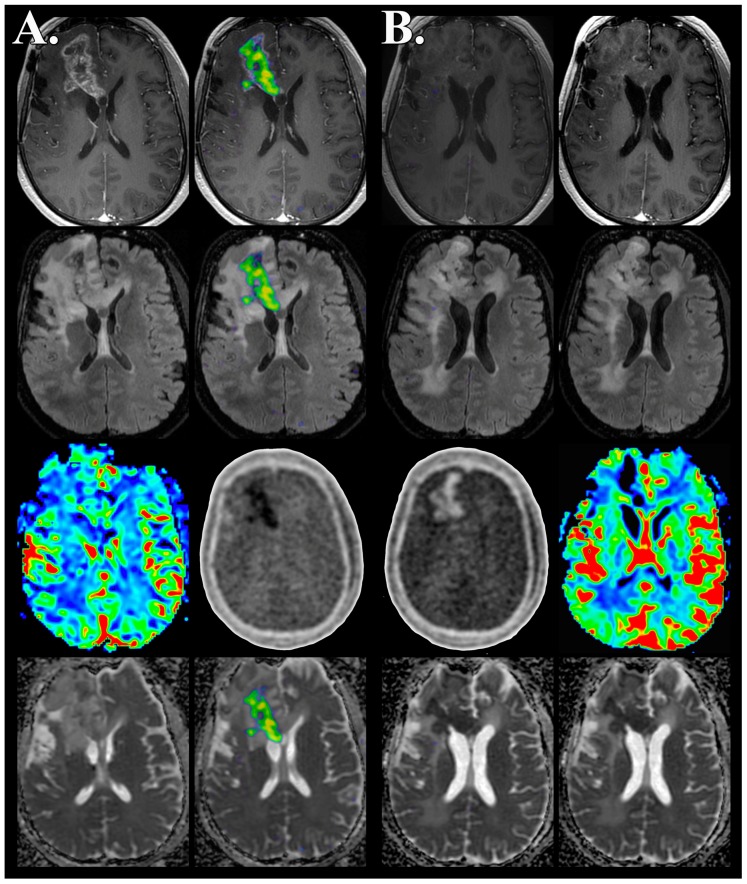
Absent FMISO hypoxic volume with associated development of reduced diffusion in recurrent high grade glioma concurrent with bevacizumab therapy. FMISO PET/MR imaging obtained simultaneously 5 days prior to (A) and 100 days following antiangiogenesis therapy (B) demonstrates progression of recurrent disease characterized by increased volume of nonenhancing mass (contrast, top row; FLAIR, top middle row) associated with the development of reduced diffusion (adc map, bottom row). Fused FMISO PET imaging (baseline, middle left; follow-up, middle right) demonstrates the resolution of tumor hypoxia concurrent with antiangiogenesis therapy. The unprocessed FMISO PET image (baseline, middle left; follow-up, middle right; bottom middle row) demonstrates the marked decrease in radiotracer accumulation below background tissue levels within regions of preserved cerebral blood volume (baseline, left; follow-up, right; bottom middle row) suggesting tissue consisting of highly cellular recurrent tumor with transformed or normalized tumor microvasculature.
